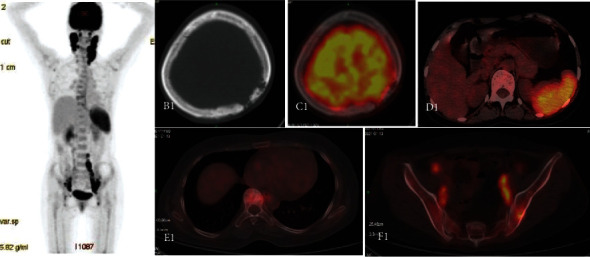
Female, 35 years old, MS-LCH (lymph nodes + bone marrow + spleen). A1 was PET MIP; B1 was CT cross-section; C1, D1, E1, and F1 were PET/CT fusion, respectively. A1 showed multiple enlarged lymph nodes observed in the bilateral neck, pelvic cavity, and retroperitoneum, with increased FDG uptake and SUVmax of 8.7. B1 showed bone destruction at the frontal bone of the left side, with no significant increase in FDG uptake (C1); D1 showed no enlargement of the spleen but diffusely increased FDG uptake when SUVmax was 5.6. E1 showed increased thoracic bone density and slightly increased FDG uptake with SUVmax of 2.9. F1 showed increased focal FDG uptake in the left iliac crest with SUVmax of 5.3. PET/CT was performed after 8 cycles of chemotherapy. A2 was PET MIP; B2 was CT cross-section; and C2, D2, E2, and F2 were PET/CT fusion. A2 showed no abnormal increase in FDG uptake, B2 showed basic repair of the left frontal bone, D2 spleen metabolism reduced to normal, E2 high-density shadow became weak, and metabolism decreased, and F2 left iliac bone lesion metabolism dropped to normal.
